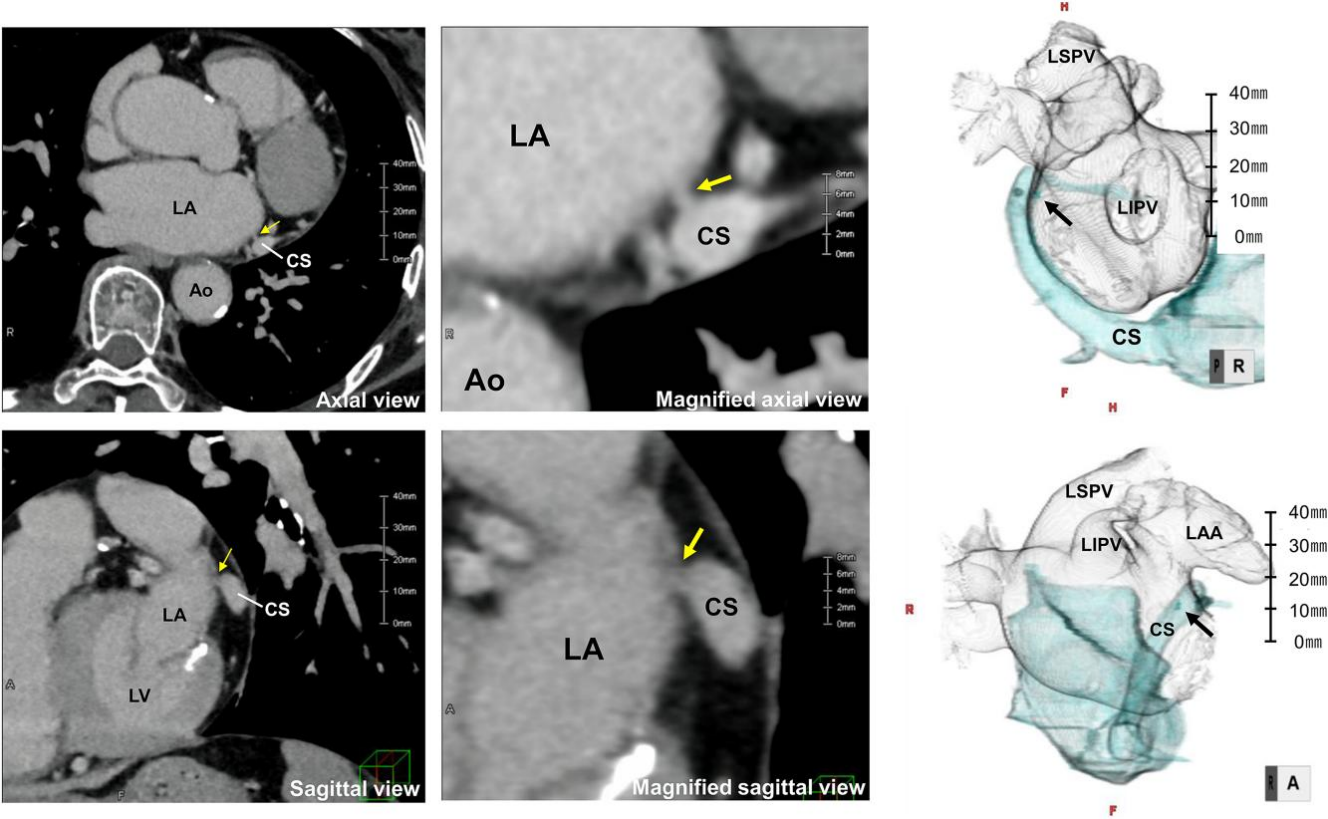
Computed tomography images including axial, magnified axial, sagittal, and magnified sagittal views, as well as a 3D reconstruction, demonstrating a connecting vein arising from the distal coronary sinus and entering the left atrium at the left atrial appendage–left inferior pulmonary vein ridge. Yellow arrows indicate the connecting vein on CT images, and the black arrow highlights the same structure on the 3D reconstruction. Scale bars are shown for reference. LA, left atrium; LAA, left atrial appendage; LIPV, left inferior pulmonary vein; LSPV, left superior pulmonary vein; CS, coronary sinus; Ao, aorta; LV, left ventricle.
